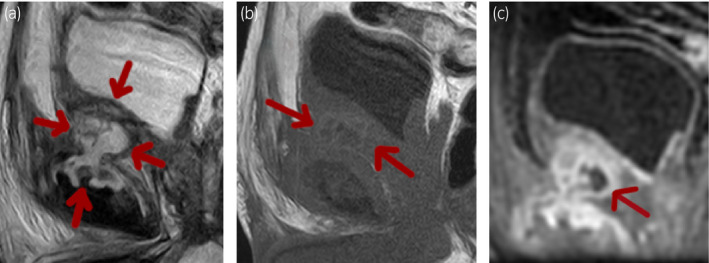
Fig. Magnetic resonance images. (a) Sagittal T2‐weighted image showing a high intensity, irregular‐shaped mass with a maximum diameter of 5.4 cm, extending from the posterosuperior aspect of the pubic symphysis to the anteroinferior aspect of the bladder (red arrows). (b) T1‐weighted image showing a mass of the same low intensity as the bladder wall. Notably, the central part of the mass exhibited a decreased signal (red arrows). (c) Contrast‐enhanced fat‐suppressed T1‐weighted image showing enhancement in the majority of the mass, except the center, which corresponded to the area of decreased intensity on unenhanced T1‐weighted imaging, thereby indicating a cystic component (red arrow).
